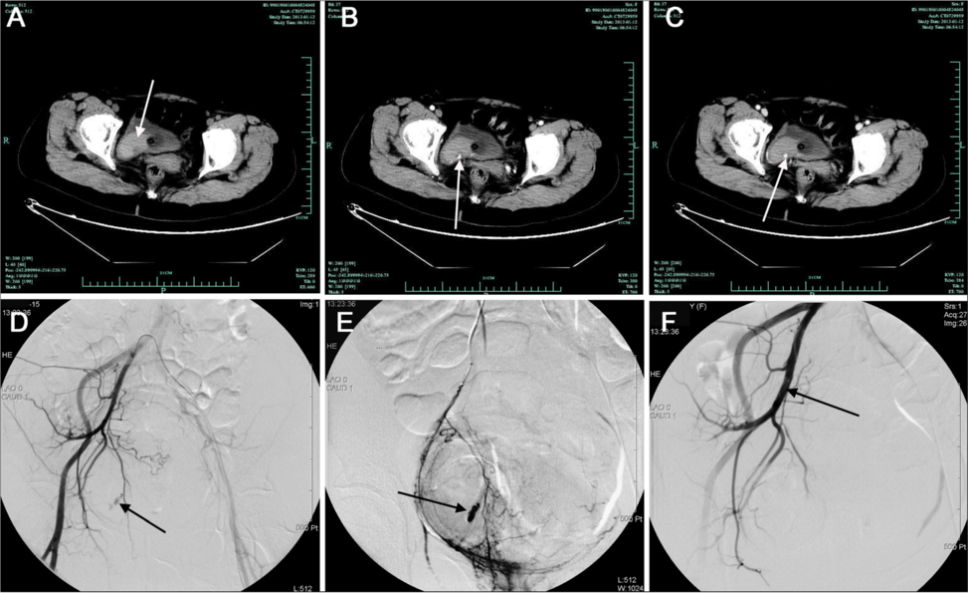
Pelvic computed tomography and angiography showed the source of the bleeding managed by transarterial embolization. (A) Noncontrast pelvic computed tomography (CT) revealed a blood clot *(arrow)* in the bladder; Contrast pelvic CT demonstrating the contrast blush suggestive of a pseudoaneurysm (arrow) (B) arterial phase CT image; (C) parenchymal phase CT image; (D, E) The pelvic angiography showing a pseudoaneurysm (arrow) of the superior vesical artery on the right side which was the source of the bleeding. (F) A successful endoluminal occlusion of the anterior trunk of the right internal iliac artery with a coil *(arrow).*
